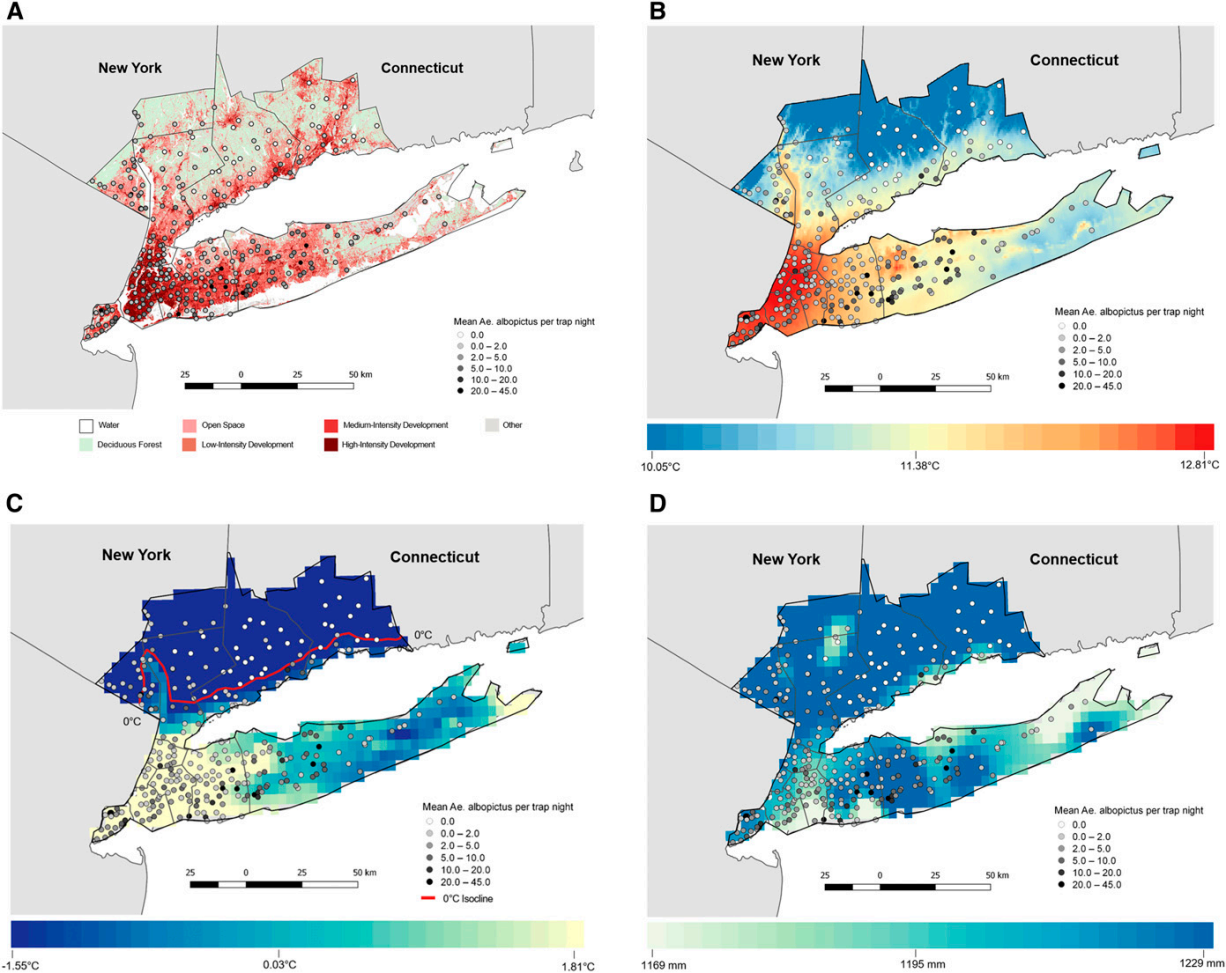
Land cover and climate across the study region, New York state and Connecticut (CT), United States. (A) Land cover classification across the study region. New York City (NYC) counties feature the greatest proportion of developed land (excluding water) (Bronx [79.44%], Kings [86.75%], New York [87.82%], Queens [88.61%], and Richmond [63.47%]), whereas Putnam (6.56%), Rockland (19.21%), and Fairfield, CT (23.04%) have the lowest. (Source: National Land Cover Database [NLCD, 2011]). (B) Mean annual temperature over the most recent three full decades (1981–2010). Temperatures are highest in NYC counties and lowest in the northern latitudes of Putnam, Fairfield, and New Haven counties. (Source: PRISM [Parameter-elevation Regression on Independent Slopes Model]. 30-year normals (1981–2010) at a 4-km spatial resolution. (C) Mean winter (December–February) temperature, 1981–2010. Again, temperatures are highest in NYC counties and the tip of Suffolk County, and lowest further inland from the Atlantic Coast (Rockland County) and the northern latitudes of Putnam, Fairfield, and New Haven counties. (D) Mean cumulative annual precipitation, 1981–2010. Across the study region, the mean cumulative annual precipitation was 1,202 mm; however, areas along the shoreline of Kings, Queens, Nassau, and Suffolk counties received less than the mean. This figure appears in color at www.ajtmh.org.
